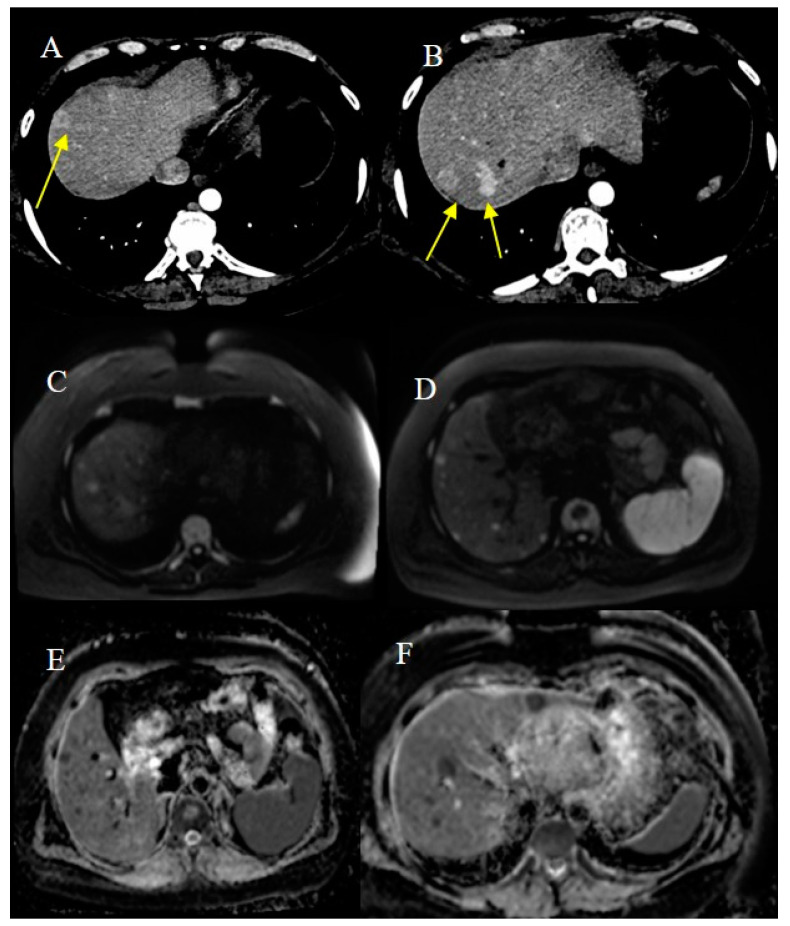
A case of hypervascular liver metastasis (yellow arrow) on contrast-enhanced CT (CECT) from an ileal neuroendocrine tumor (NET) is shown in images (A,B). In the MRI diffusion-weighted imaging (DWI) sequence with a higher b value, more liver metastases are visible as hyperintensity signals in images (C,D), with lower apparent diffusion coefficient (ADC) values in images (E,F).
